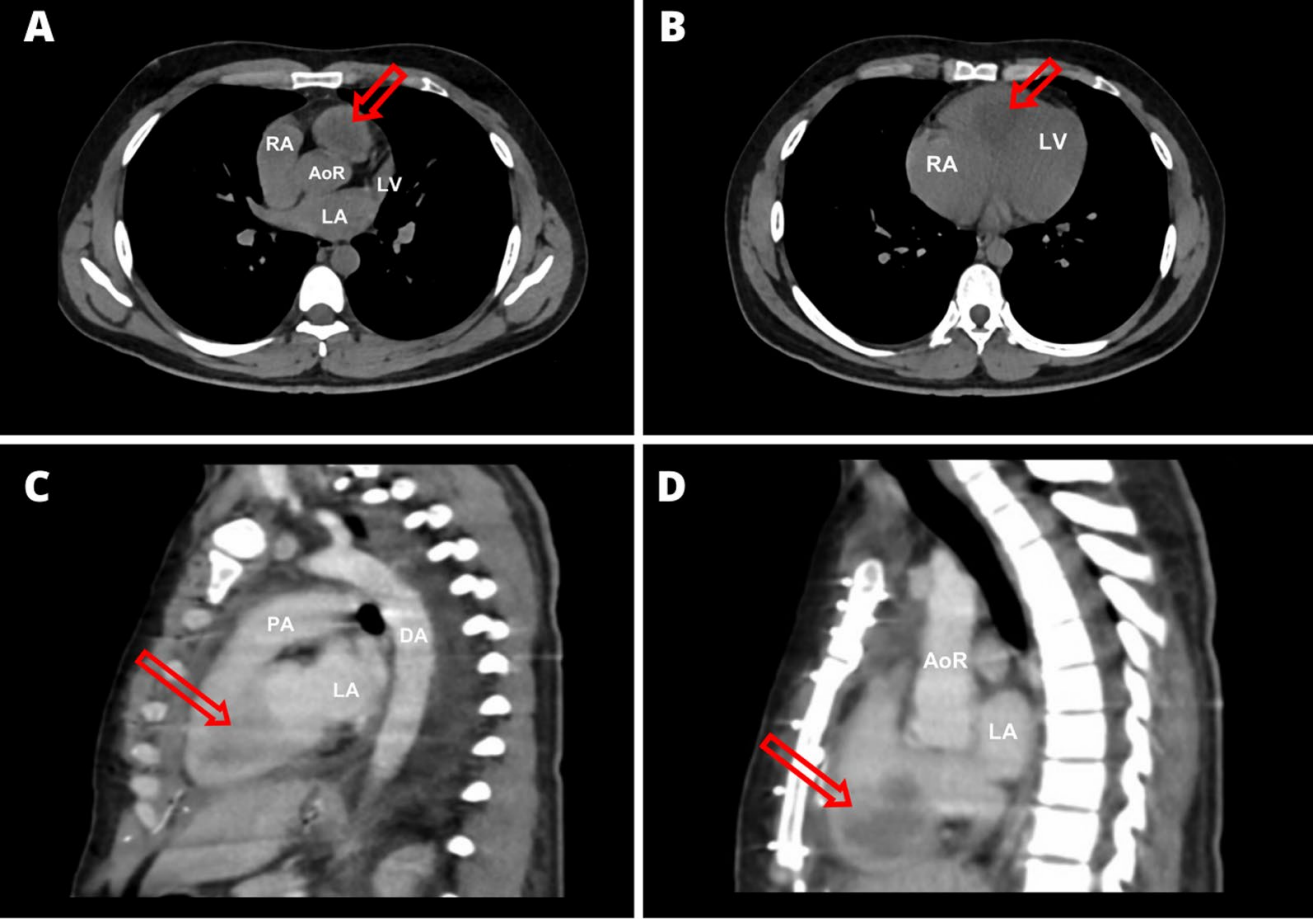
Axial CT scans without contrast A and B depict a mass occupying the right ventricular outflow tract (arrow). Sagittal CT scans with contrast C and D reveal a mass in the right ventricle (arrow). AoR: root of the aorta, RA: right atrium, LA: left atrium, LV: left ventricle, DA: descendent aorta, and PA: pulmonary artery
